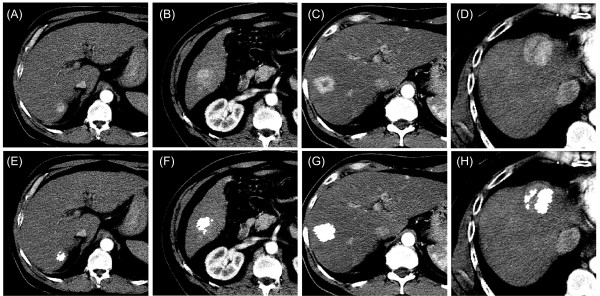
Highlighting of enhanced HCC in real CT-images. Input images
